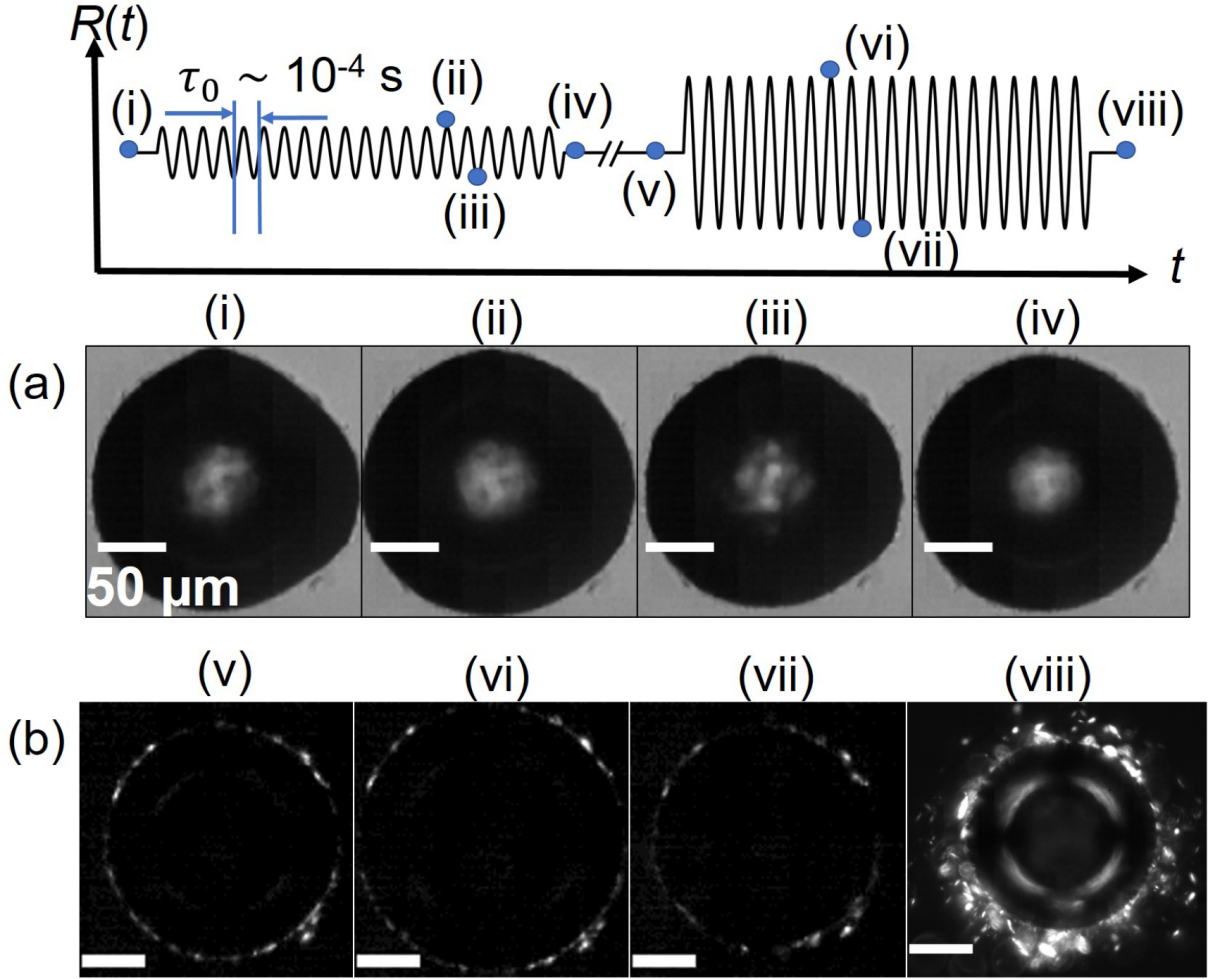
Crystal expulsion after ultrasound-driven bubble oscillations.
